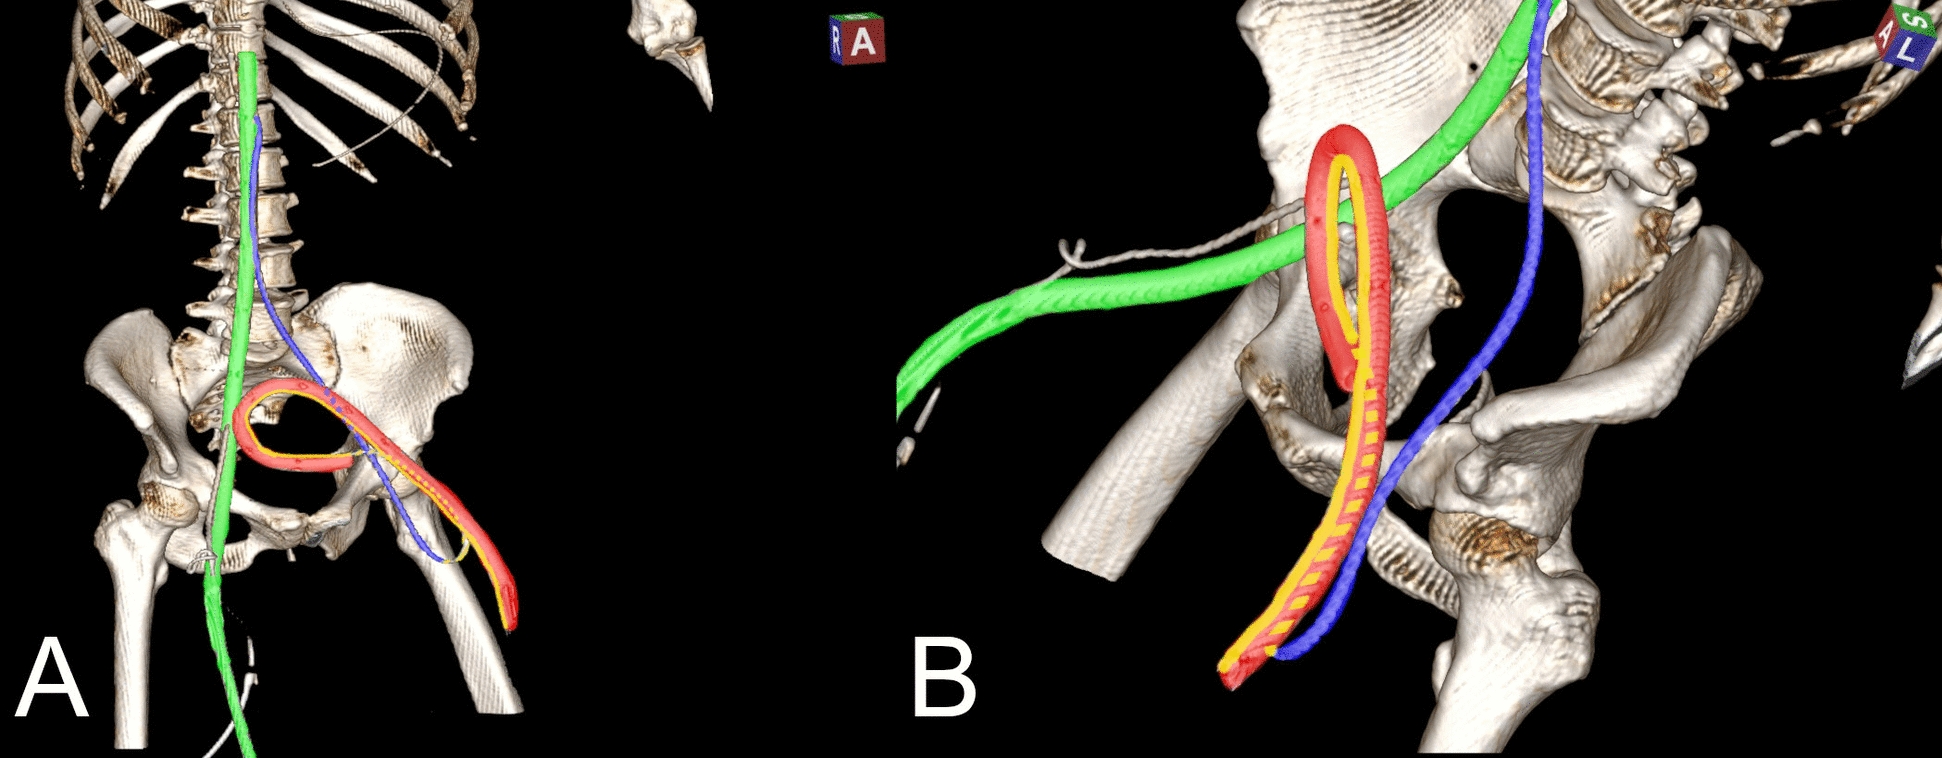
Three-dimensional reconstruction of the computed tomography scan. Panels A and B show two different view angles of the same picture. The computed tomography findings revealed that the right femoral cannula was properly placed intravascularly (green), while the left cannula was in the wrong location, intraperitoneally in the Douglas space (red). Interestingly, the guidewire of the misplaced cannula was in the correct intravascular position, and its trajectory can be traced from the femoral vein to the inferior vena cava (blue line). However, owing to wire kinking (as seen at the blue–yellow crossing point), an extravascular portion of the wire was also visible (yellow line, dashed in its path outside the cannula). Consequently, the cannula followed the wire’s extravascular path and entered the peritoneum
